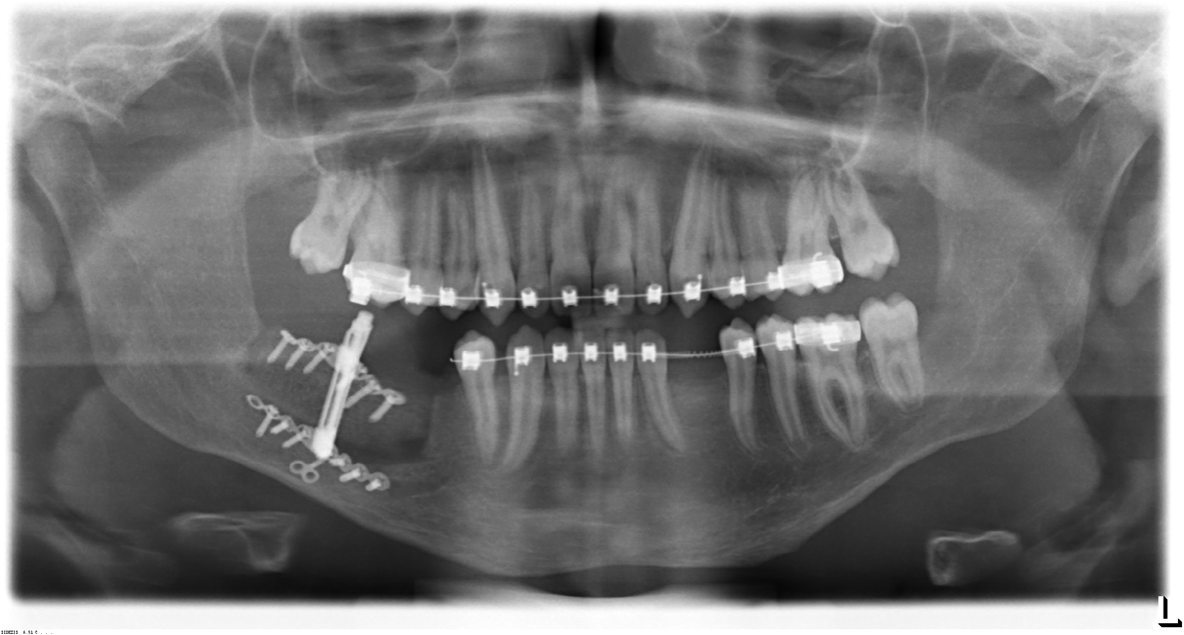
Orthopantomogram after insertion of the distractor and distraction to the maximal height. Subsequently, the consolidation phase followed
